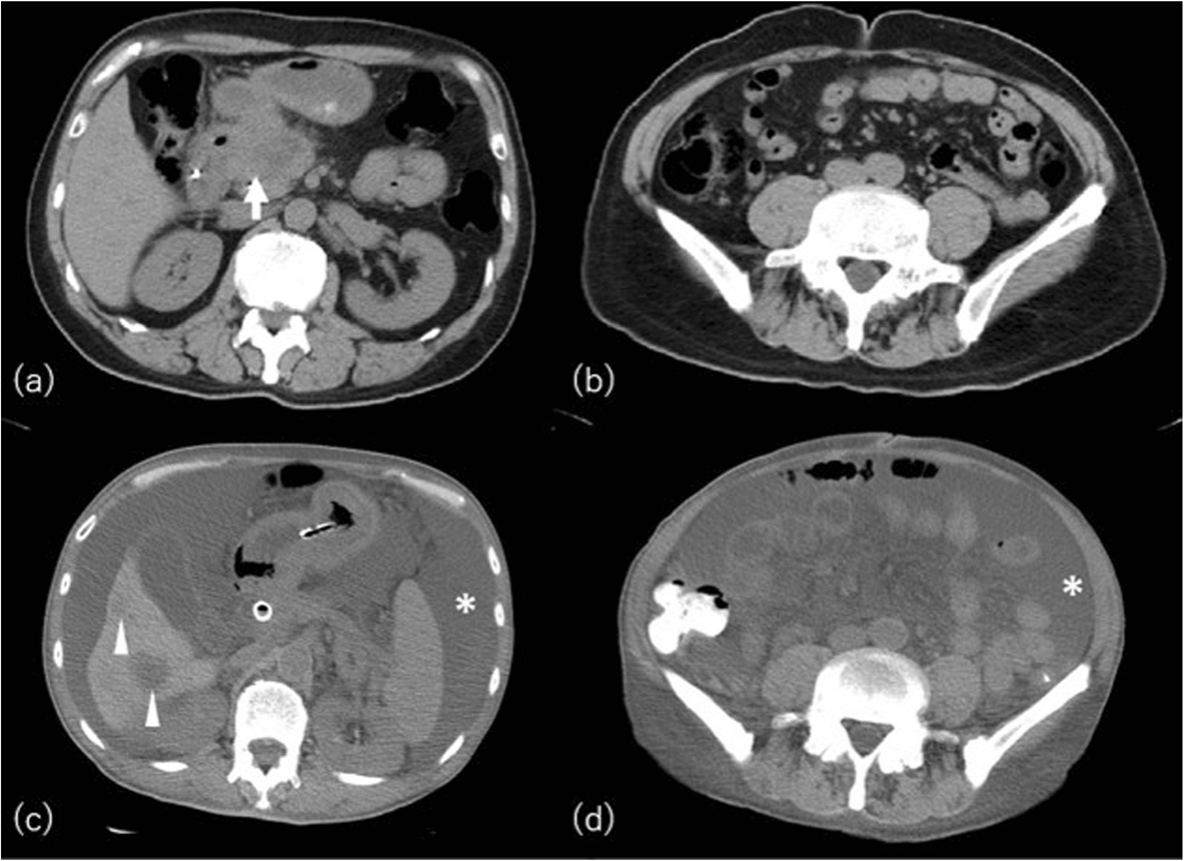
A 68-year-old man with pancreatic cancer. Initial CT in our hospital (a, b) shows massive low-density mass in the head of the pancreas (arrow). CT performed 14 months later (2 months before death) (c, d) shows depletion and increased attenuation of subcutaneous and visceral adipose tissue. Hepatic metastases (arrowheads) and copious ascites (asterisks) are also observed
